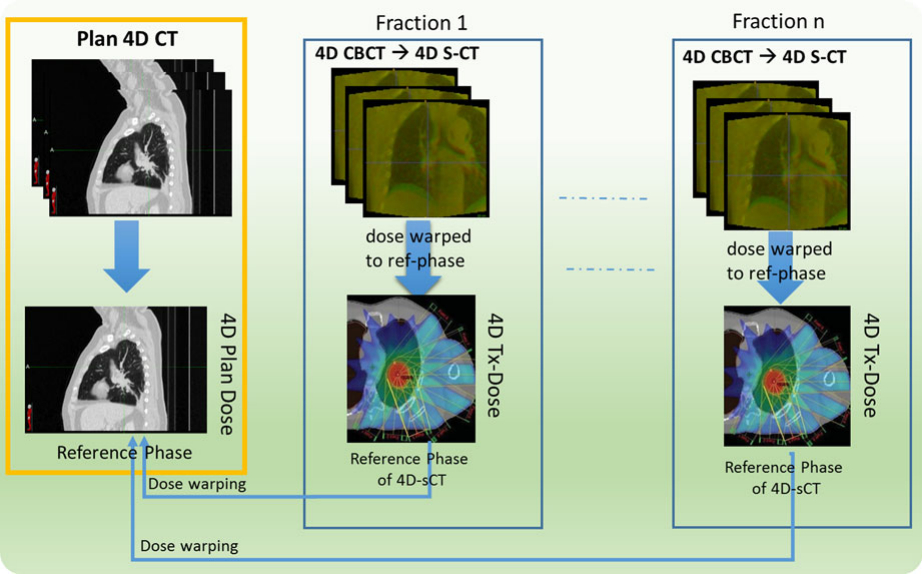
4D treatment dose reconstruction based on 4D‐CBCT for lung cancer patient.
